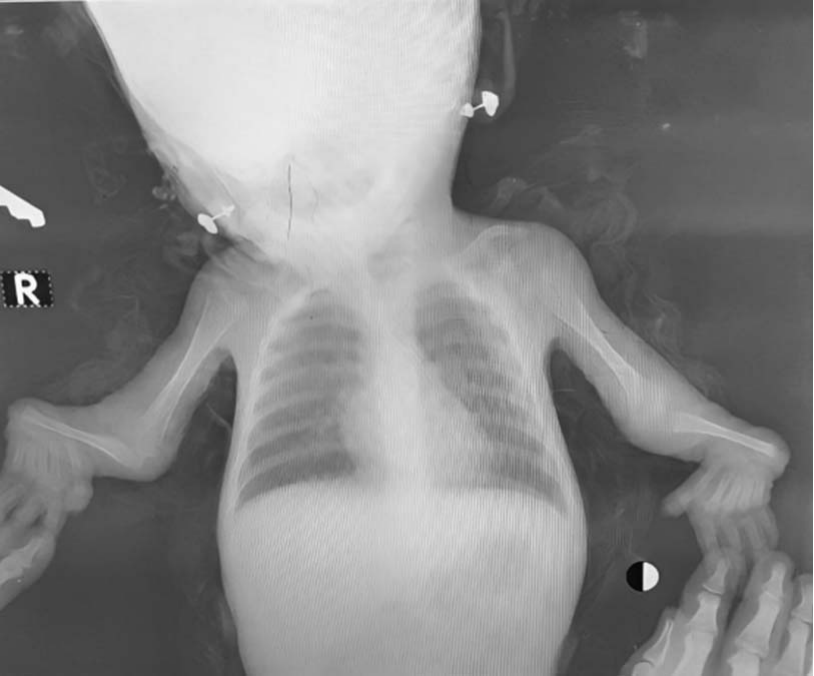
X-rays of the upper limbs demonstrated bilateral absence of the radii.
